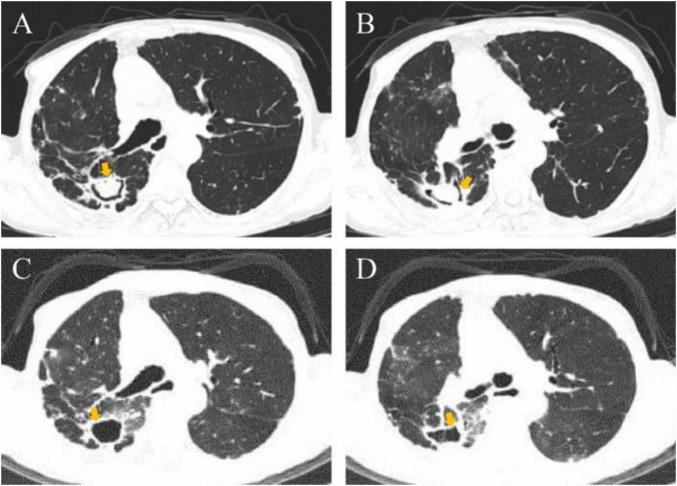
Chest CT images of the patient before and after bronchoscopy. (A,B) The manifestation of *Aspergillus* ball in the posterior segment of the right upper lobe and the dorsal segment of the right lower lobe before bronchoscopy in July 2025. (C,D) The *Aspergillus* ball disappeared after the bronchoscopy operation.
